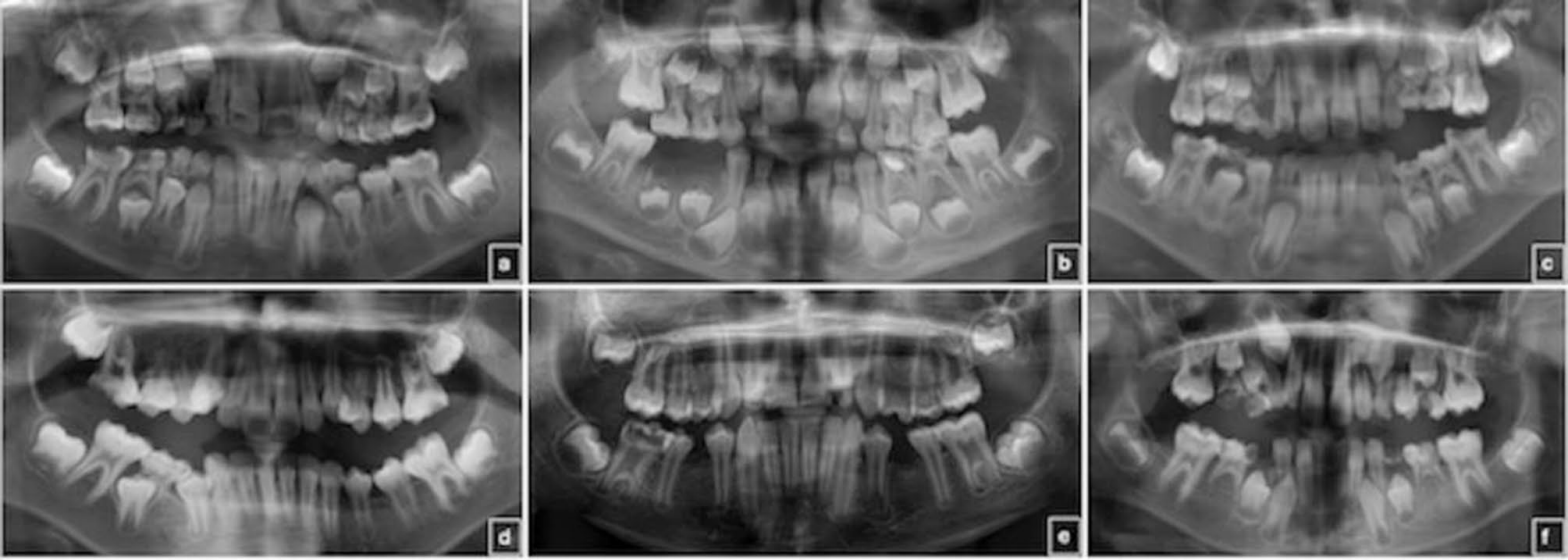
Representations from panoramic radiographs of children diagnosed with molar incisor hypomineralization and developmental dental anomalies. Macrodontia in maxillary central incisors is in a; ectopic eruption of maxillary permanent first molars is in b; infraocclusion of primary molars in left and right maxillary and left mandibular posterior regions is in c; dilaceration of the left mandibular permanent first molar root is in d; taurodontism in all permanent first molars is in e; and oligodontia is in f
